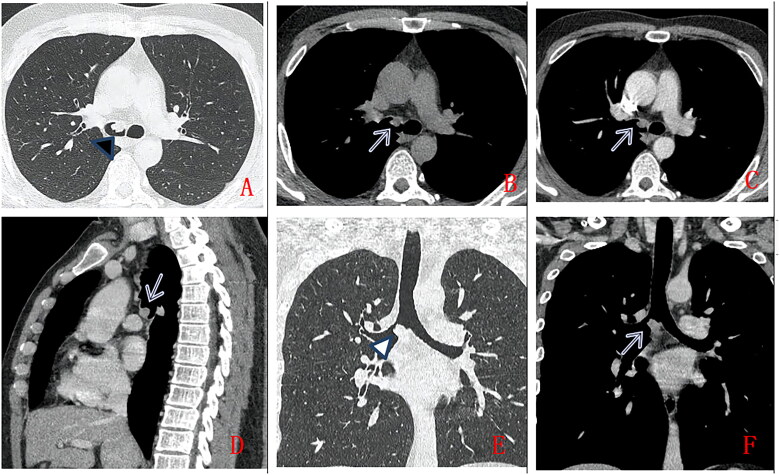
A 36-year-old man, with no clinical symptoms, chest CT scan without contrast (A.axial lung window, B.axial mediastinum window) and with contrast (C.axial, D. Sagittal, E.coronal lung window, F. coronal mediastinum window). A solid nodule in right main bronchus (as indicated by arrow), with smooth margin,uniform density, moderate enhancement. Pathologically confirmed low-grade PMEC after surgical operation.
